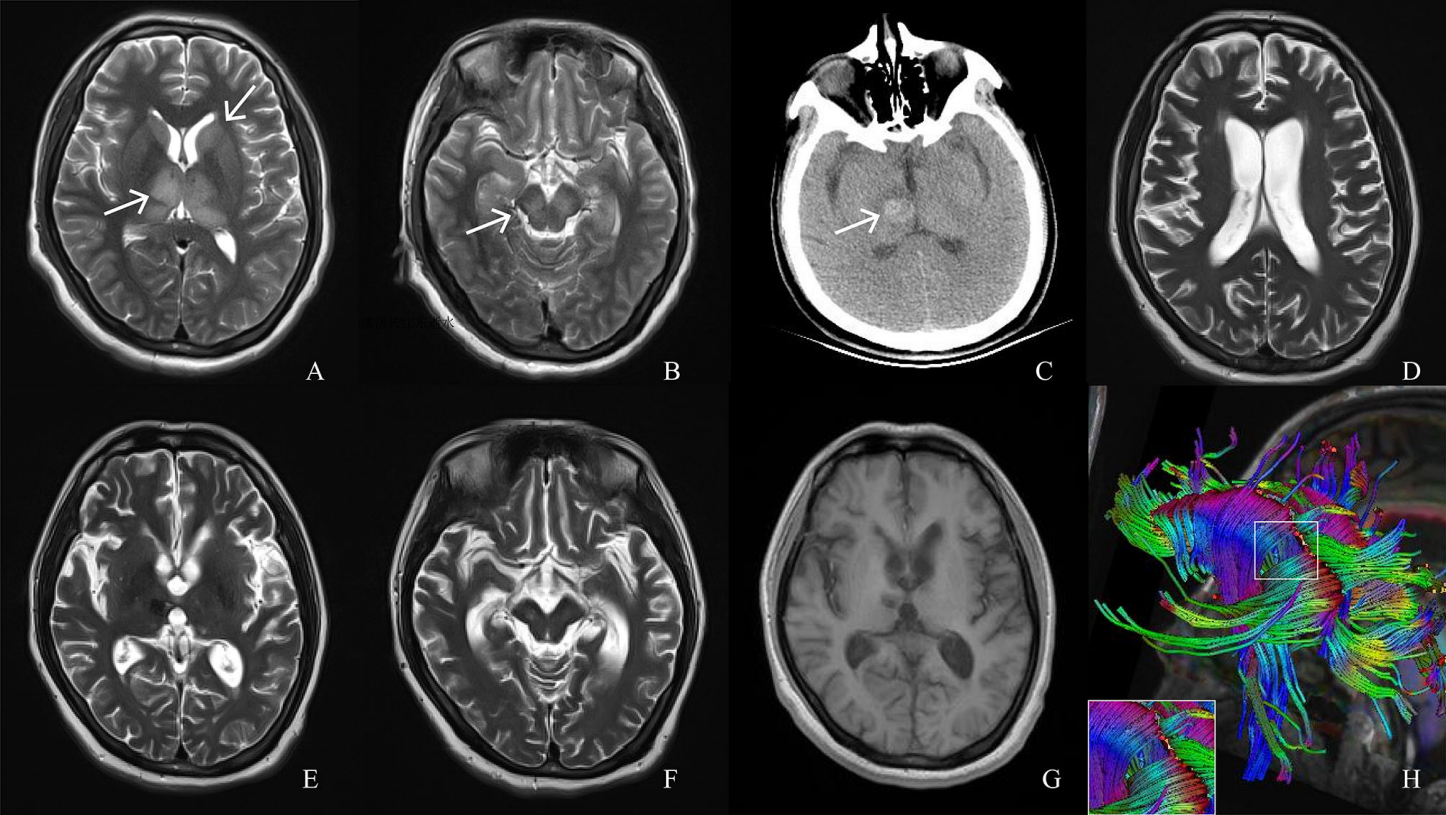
Magnetic resonance imaging (MRI), computed tomography (CT) and diffusion tensor imaging (DTI) scans of the patient. (A, B) T2 MRI on day 6 of onset shows hyperintense signals in the thalamus and caudate nucleus (white arrows) and cerebral peduncles (white arrows). (C) CT performed on day 19 of onset, when the patient fell back into a coma, shows right thalamus hemorrhage (white arrow). (D–F) T2 MRI re-examination at month 6 of the course of disease shows extensive brain atrophy and the lesion area was smaller than before. (G) T1MRI examination at month 14 of the course of disease shows brain atrophy. (H) DTI at month 14 of the course of disease shows partially broken corpus callosum tract.
